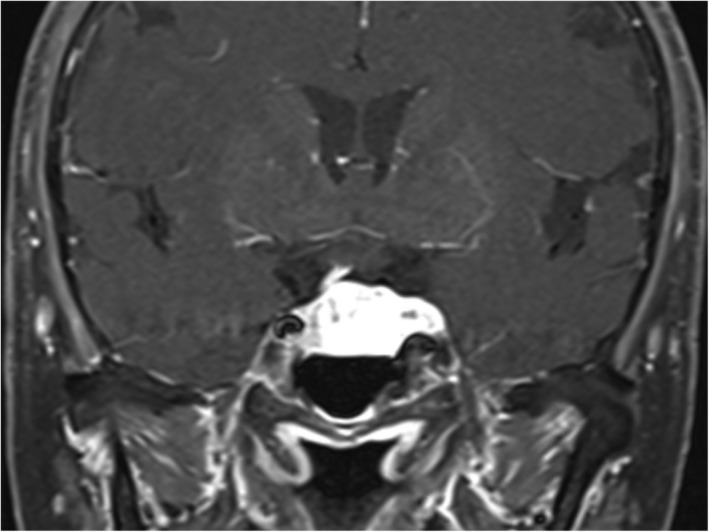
Coronal T1‐weighted contrast MRI showed a homogeneous enhancement of sellar and suprasellar mass
